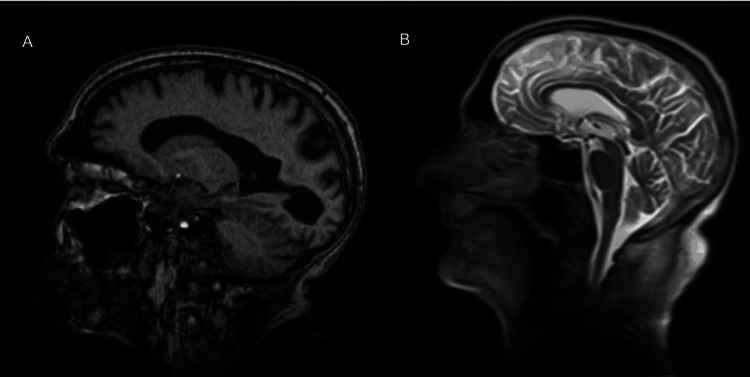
Sagittal magnetic resonance images: T1-weighted sequence (A) and T2-weighted sequence (B), showing no evidence of lesions in the basal ganglia.
